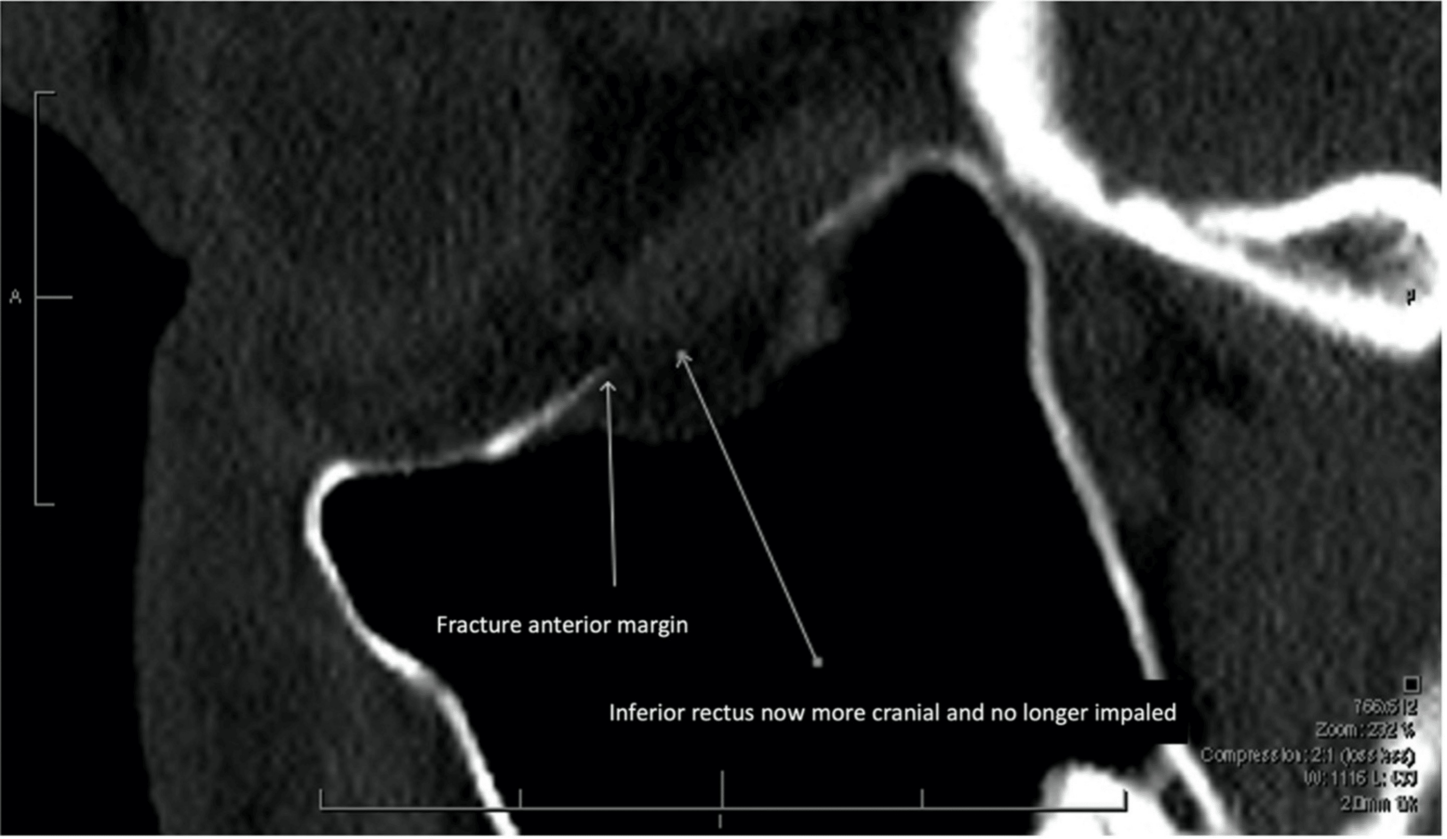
Sagittal CT Orbits (Bony Windows), Following Forced Duction Testing for Resolution of Inferior Rectus Impingement. The inferior rectus is now more cranial and no longer impaled by the anterior margin of the fractured bone.
